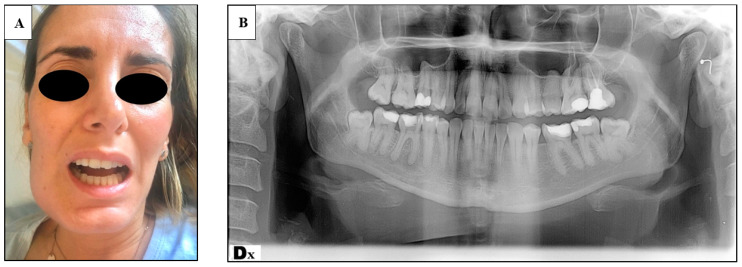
(A) Frontal view of the patient presenting submandibular swelling and trismus; (B) orthopantomography of the patient showing periapical abscess of the 4.6 tooth.
